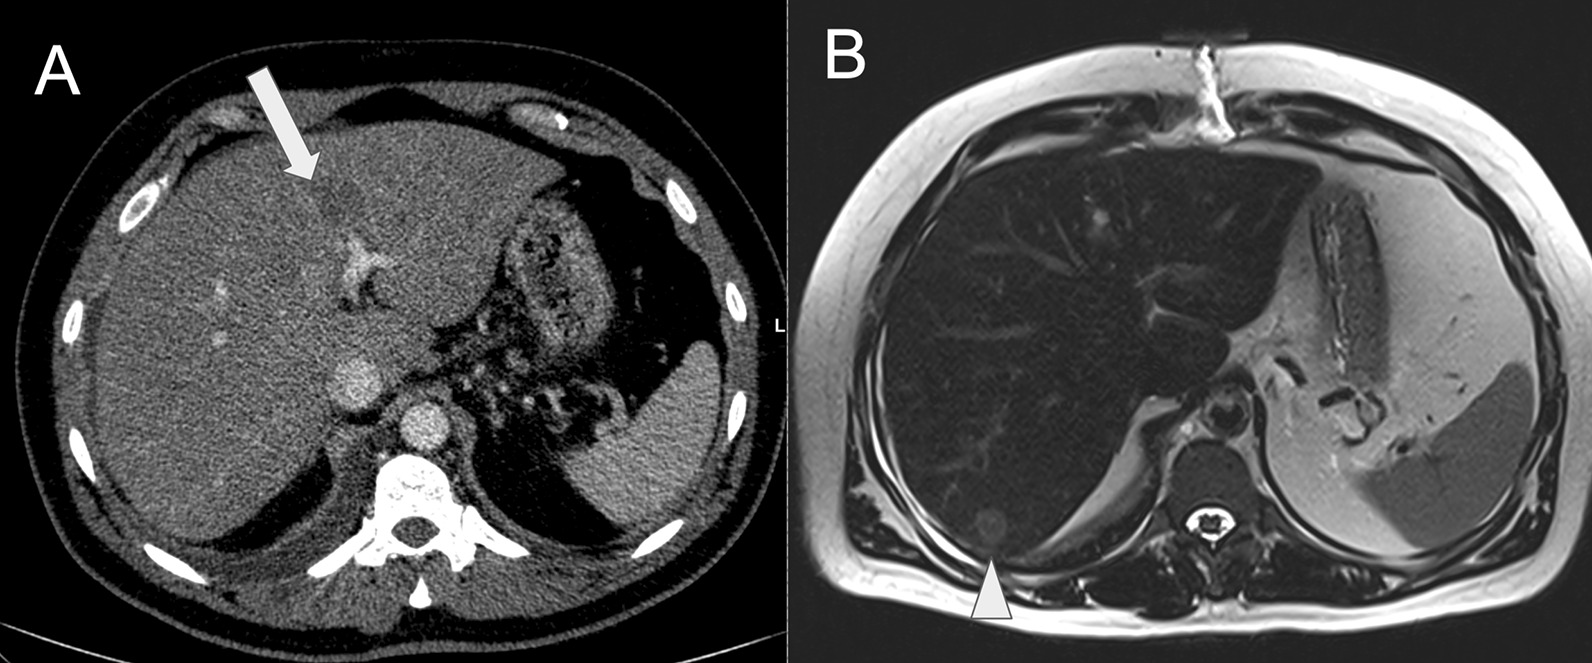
A Contrast-enhanced abdominal computed tomography showing an enlarged and heterogeneous liver, with ill-defined hypoattenuating indeterminate lesions (arrow). B Abdominal magnetic resonance (T2-weighted image) depicting a suspicious intermediate signal solid nodule (arrowhead) on right hepatic lobe
